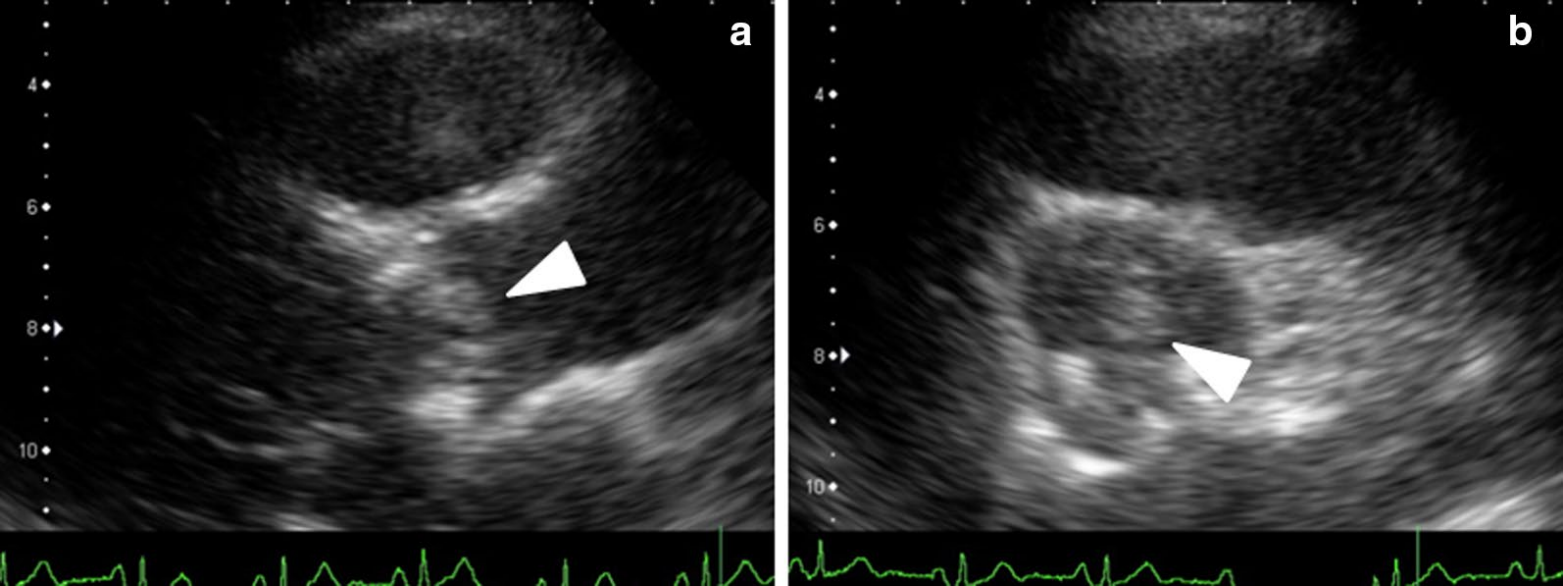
a 2D echocardiogram. Apical long-axis view shows a large vegetation (arrow), measuring 16 mm × 9 mm. b Short-axis view
